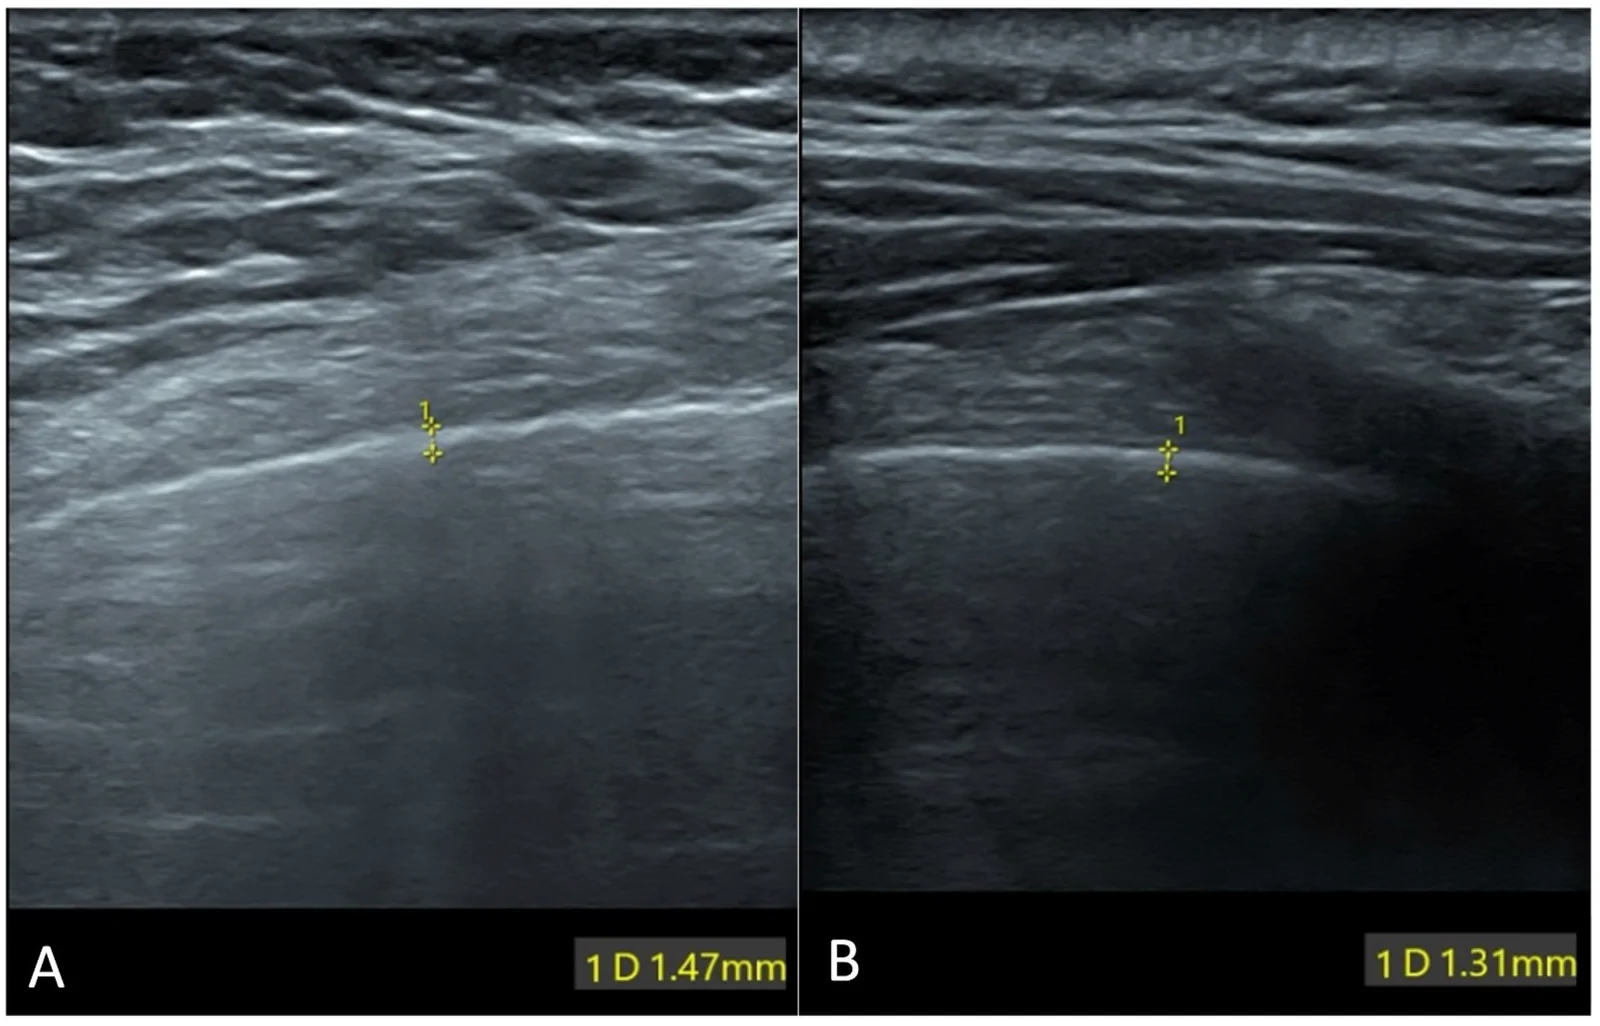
Transthoracic ultrasonography images of the left midclavicular zone before and after treatment in the same patient. (A) Baseline B-mode scan showing an irregular pleural line. (B) Week-16 B-mode scan at the same location, showing reduced pleural-line thickness and a regular pleural line. The yellow caliper markers indicate the boundaries used for pleural-line thickness measurement, and the numerical value displayed in the lower right corner indicates the measured thickness in millimeters.
